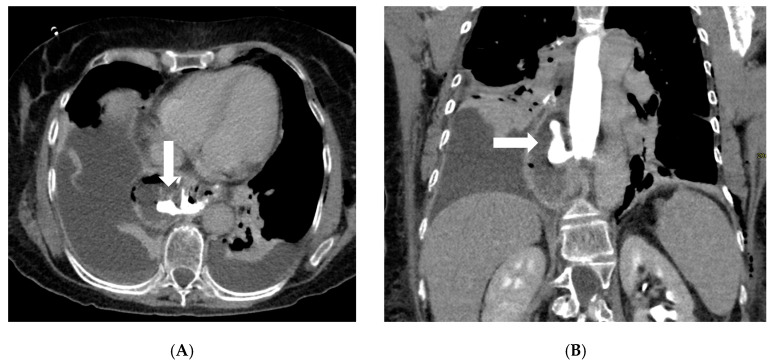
Axial (A) and coronal (B) post-contrast CT scans in a patient on postoperative day 3 following gastric bypass with esophagojejunostomy positioned in the mediastinum (history of sleeve gastrectomy). An esophagojejunal feeding tube is in place. To the right of the esophagojejunal anastomosis, a hypodense collection with internal air components is visible. After oral administration of water-soluble contrast, a posterior dehiscence of the anastomosis is demonstrated, with extraluminal contrast leakage accumulating within the previously described collection (arrows). The copyright of this figure belongs to the authors.
